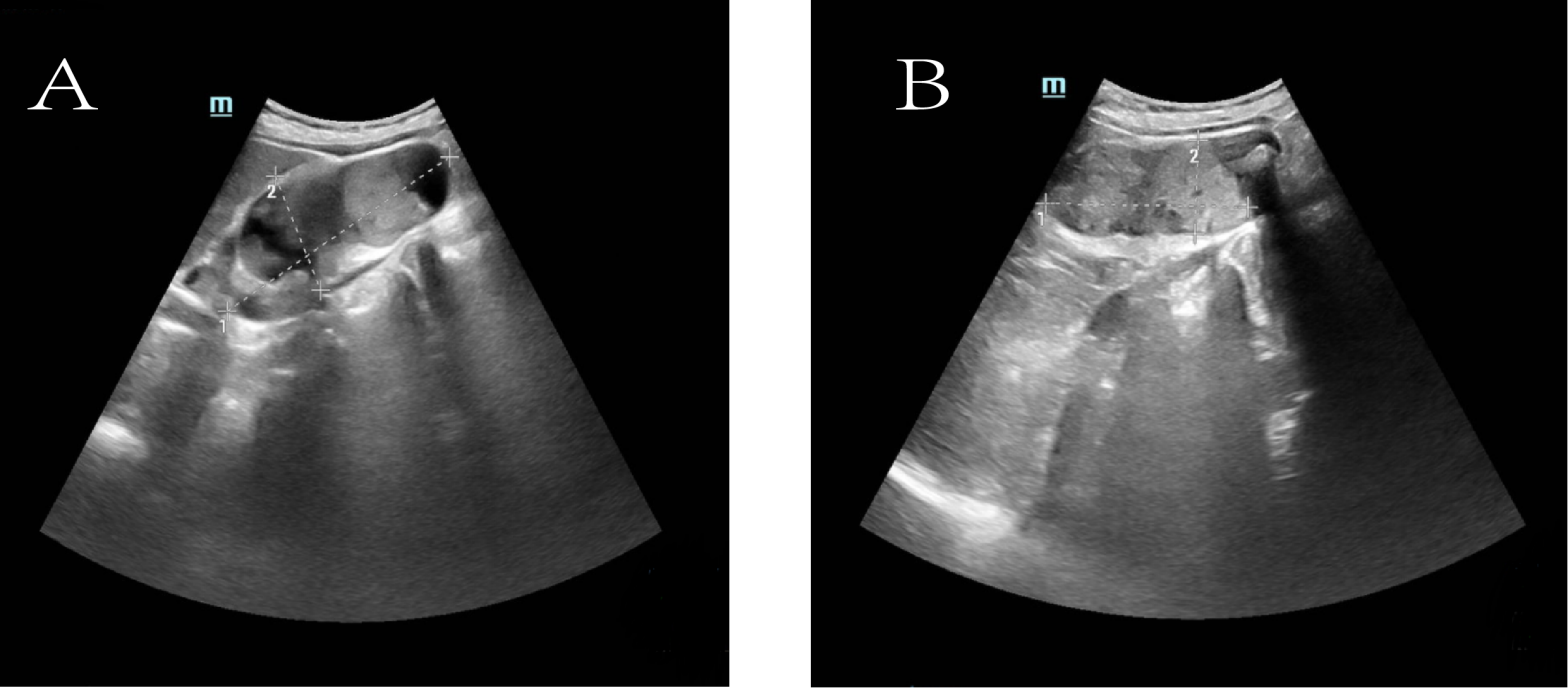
Ultrasonographic images of the gallbladder. (A) The gallbladder is enlarged (measuring approximately 9.7 × 4.2 cm) with a non-thickened wall (delineated by dashed line). Multiple hyperechoic foci are observed within the lumen, accompanied by dense posterior acoustic shadowing. (B) A medium-echoic mass, measuring approximately 7.0 × 3.1 cm, is visible within the gallbladder lumen (outlined by dashed line).
